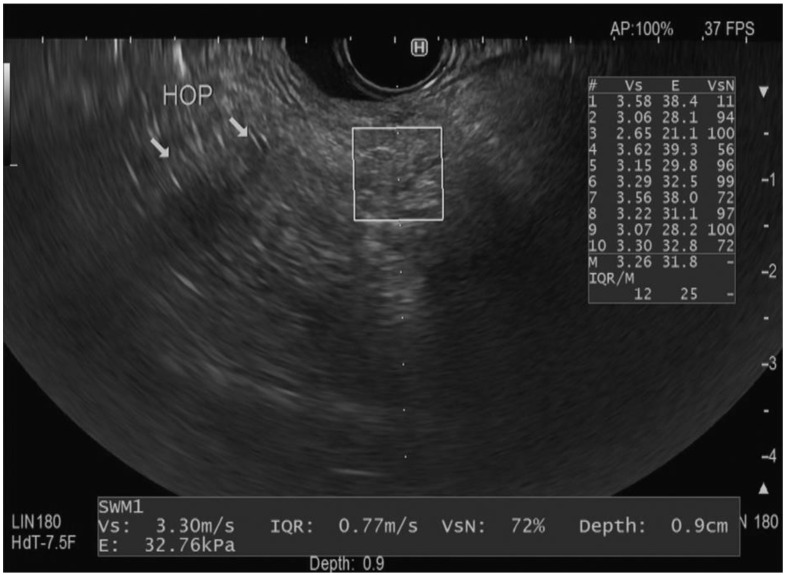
Endoscopic ultrasound view of the shear wave elastography technique in a region of interest (square) in the head of the pancreas with measurements including the velocity (Vs), interquartile range (IQR), reliability (VsN), elasticity (E), and depth [10]. The arrows reflect the pancreatic parenchyma in the head of the pancreas.
